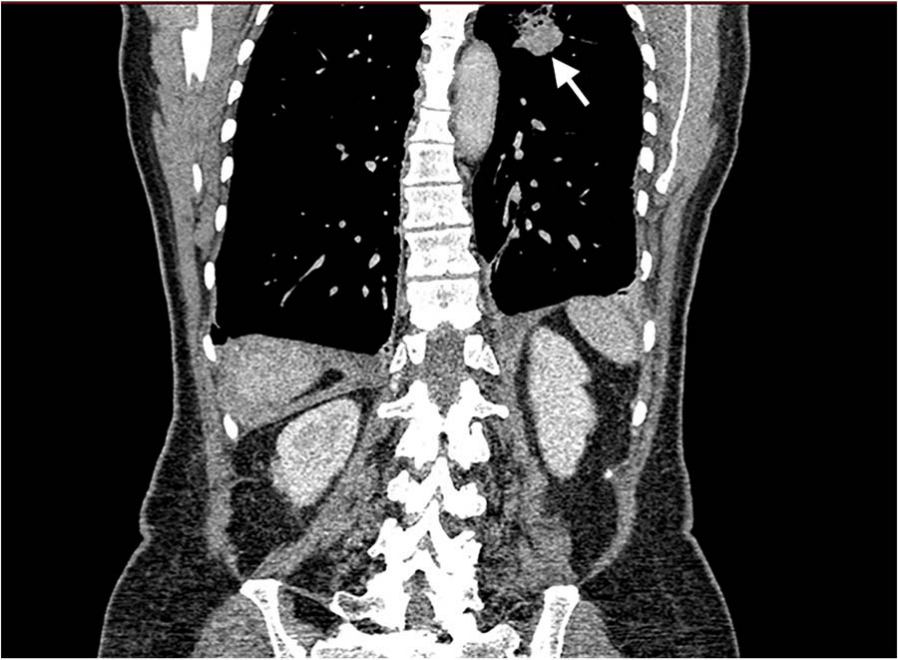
Coronal CT image of the lungs showing the cavitating mass suspected to be an abscess.
